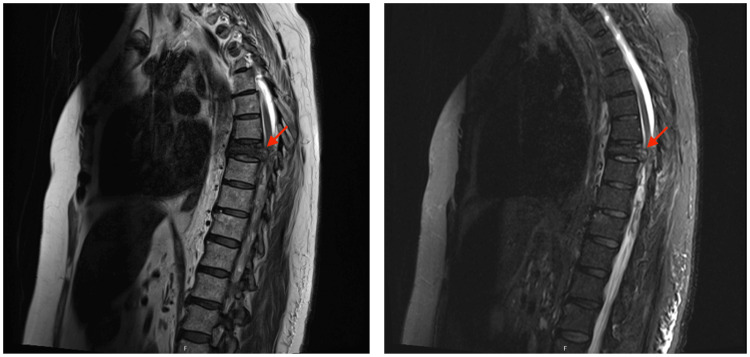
Magnetic resonance imaging (MRI) of the dorsal spine showing an expansive lesion, centered on the T8 vertebral body, causing the compression of the spinal cord (red arrows).
